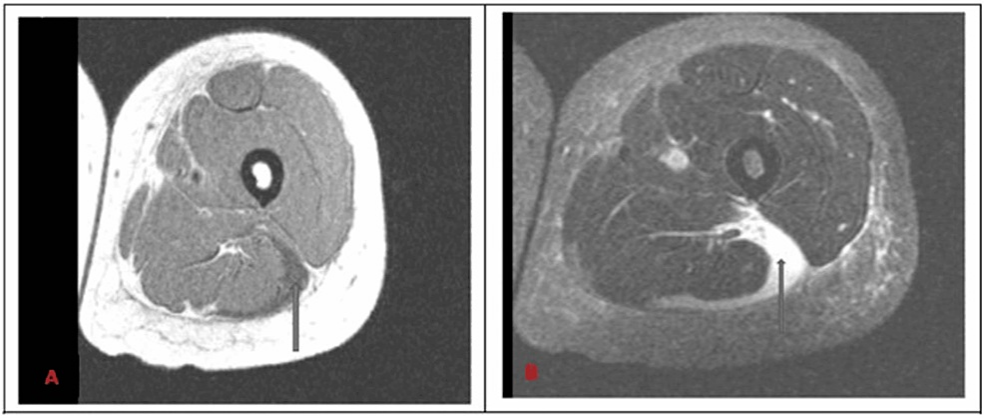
Axial T1 (A) and T2(B)-weighted MRI of left lower extremity showing large hematoma/fluid tracking in the fascial planes surrounding lateral biceps femoris muscle. The blue arrows are pointing to the hematoma
